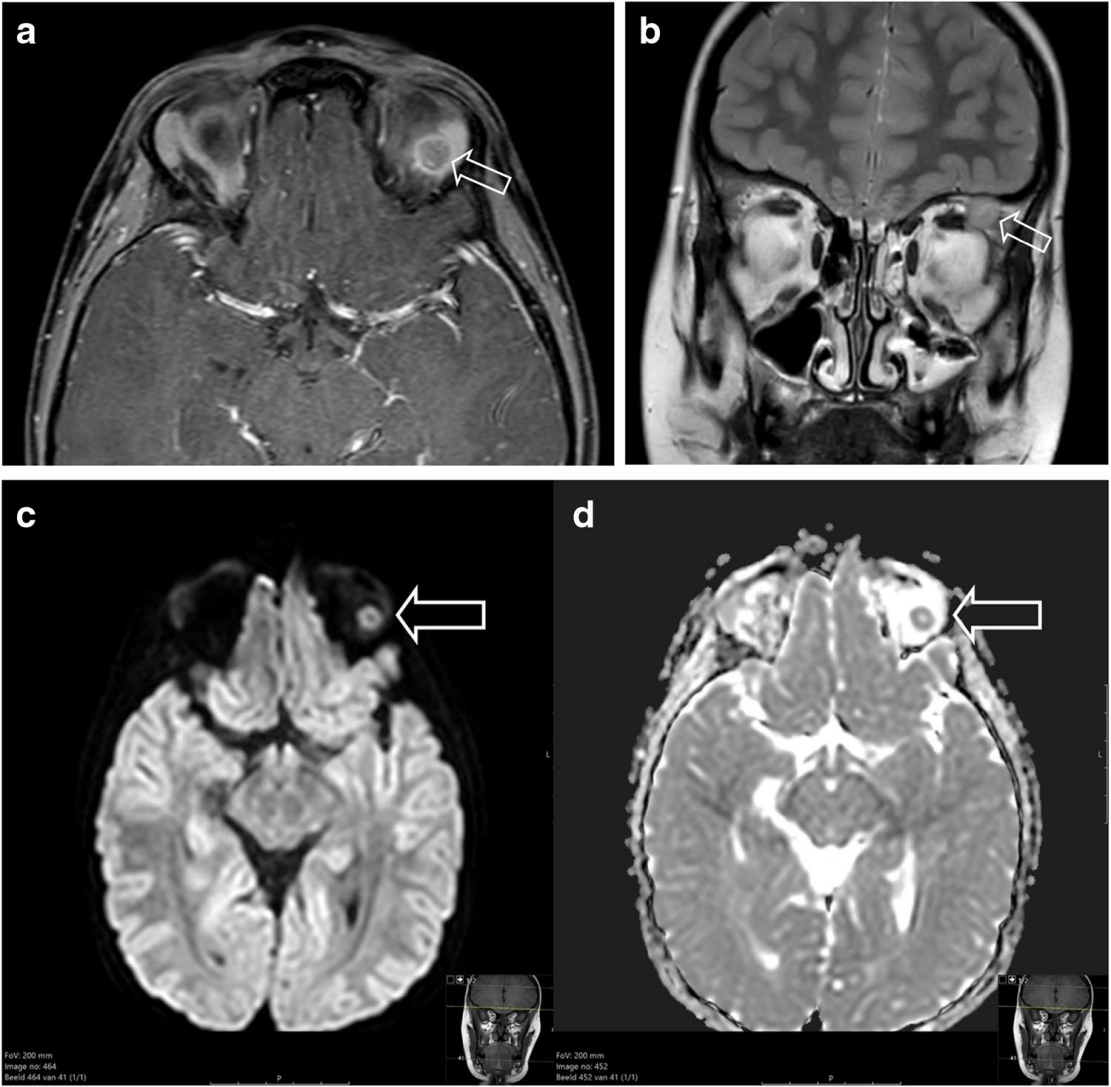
Follow-up magnetic resonance imaging of a primary orbital rhabdomyosarcoma in a 6-year-old boy (patient 1), 1 year after initial diagnosis. Axial T1 (a) and coronal T2 turbo spin echo (b) images following gadolinium show tumour recurrence (*arrows*). Axial diffusion weighted, (b-value 800) (c) and axial apparent diffusion coefficient mapping map (d), show a focus of diffusion restriction in keeping with recurrence (*arrows*)
